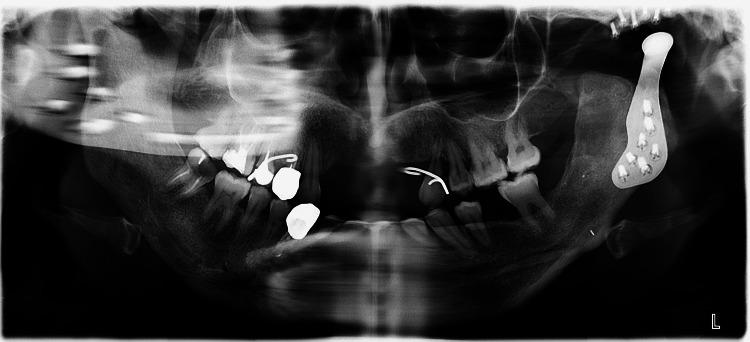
Panoramic radiograph of the initial situation
